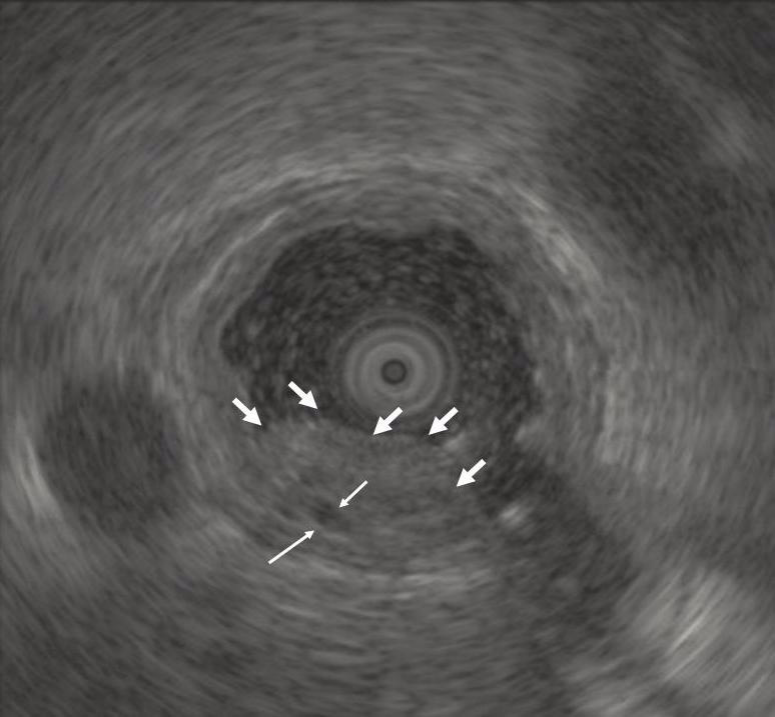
Endoscopic ultrasonography with Olympus UM2000 (Olympus Optical Company, Tokyo, Japan), demonstrates that the minute cancer was noninvasive carcinoma (carcinoma *in situ*) and a 2-mm diameter blood vessel (thin white arrows), feeding the esophageal varices, pierces the lesion. Thick white thick arrows indicate the minute cancer.
